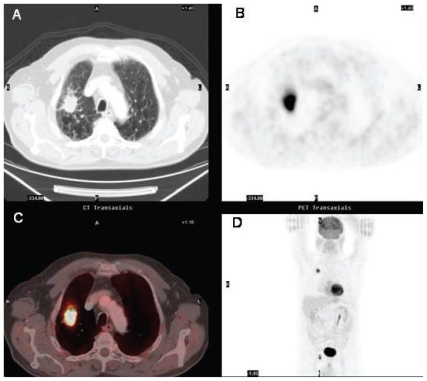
70 year-old man referred for evaluation of a pulmonary solitary nodule evaluation. Axial CT scan shows a 2.8 cm pulmonary lung in the right upper lobe with spiculated margins and emphysema. ^18^FDG PET and ^18^FDG PET/CT fusion images reveal increased ^18^FDG uptake in the nodule. The lesion corresponded to a squamous-cell carcinoma.
